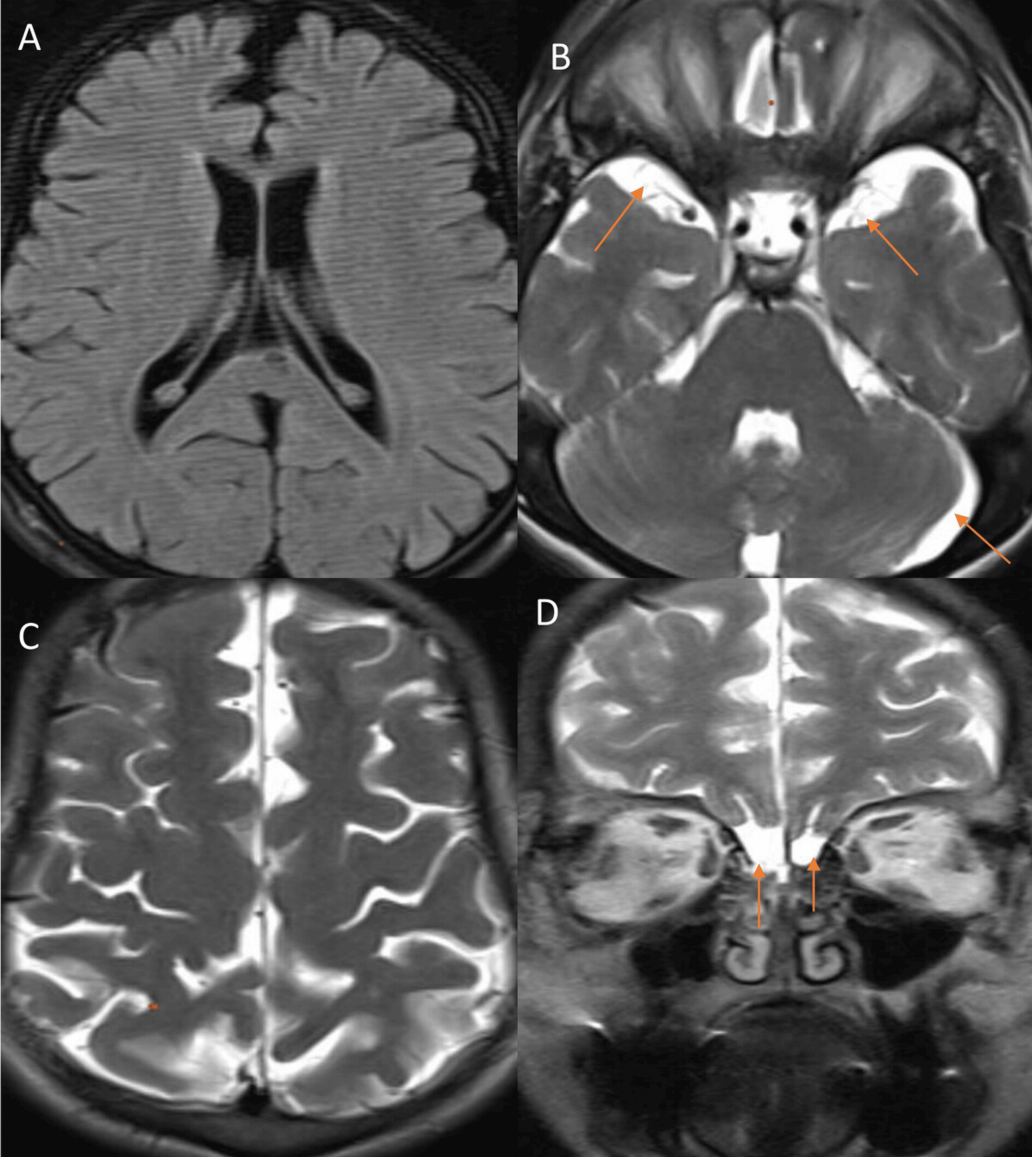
(A-C) Axial sections of the brain. (A) T1-weighted image (T1WI) shows prominent cortical sulci and ventricular system, suggestive of generalized cerebral brain atrophy. (B) T2-weighted image (T2WI) shows prominent subdural cerebrospinal fluid (CSF) spaces in the temporal region and left cerebral hemisphere (red arrows). (C) Craniosynostosis shows increased anteroposterior diameter and reduced transverse diameter, resulting in dolichocephaly. (D) The coronal section shows atrophy of the olfactory bulbs (red arrows).
